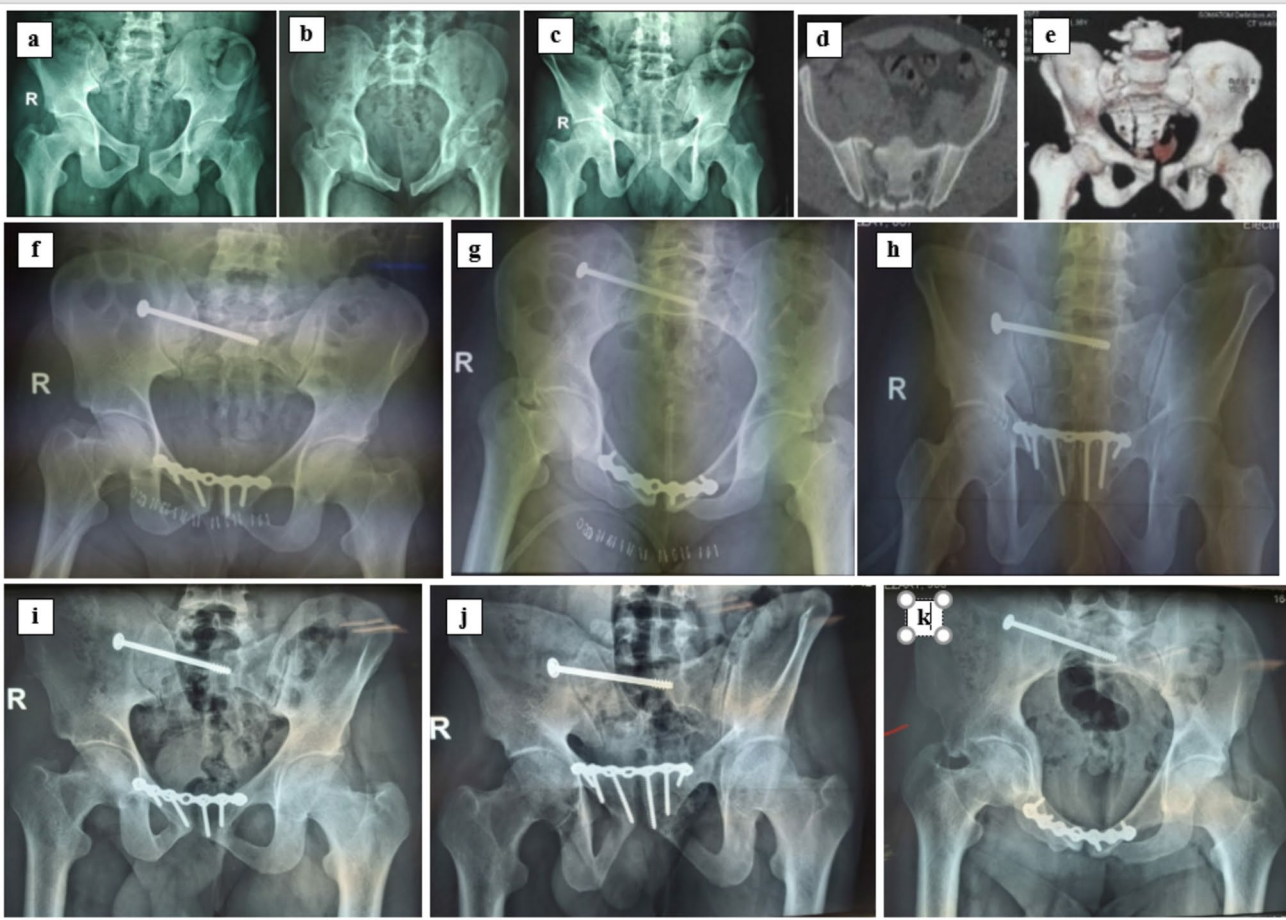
A 38-year-old male RTA. a-e preoperative radiographs and computed tomography (CT) scan showing Tile C1-3 injury (VS Fracture Sacrum). F-H postoperative radiographs show posterior ring fixation with a single SI screw and anterior ring fixation via a single superior symphyseal plate. I-K radiographs taken 14 months postoperatively
